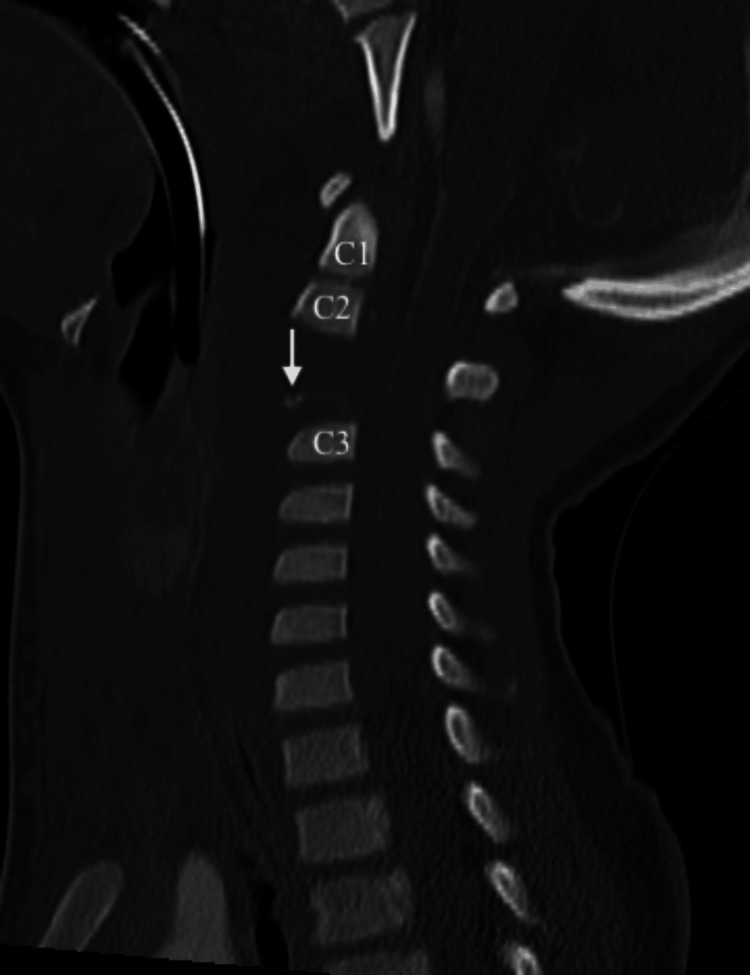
Sagittal view of CT C-spine demonstrating C2-C3 distraction injury with avulsion fracture of C2 with 1.1 cm distraction of the base of C2
